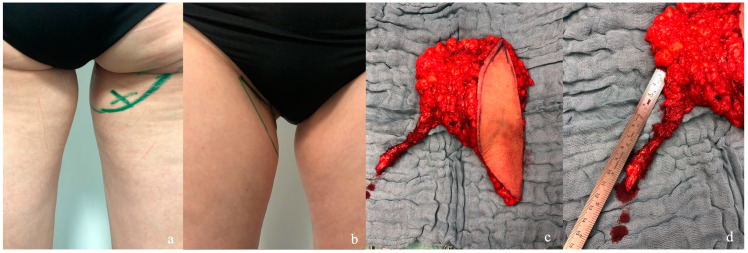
(a) Preoperative marking after location of the dominant perforator by duplex ultrasound, dorsal view. (b) Ventral view. (c) Harvested flap including a small paddle with perforator located outside. (d) Average pedicle length in our cohort was 10.5 cm ± 1.4 cm. Image is reproduced from Varnava C. et al. [9].
